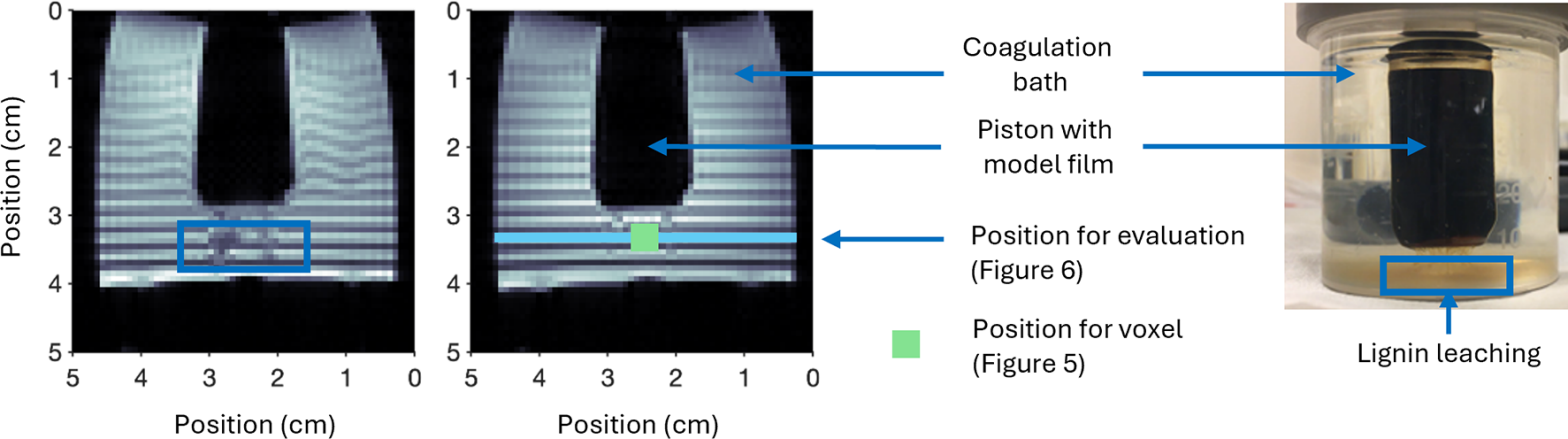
(Left) Two sagittal MRI images with saturation
